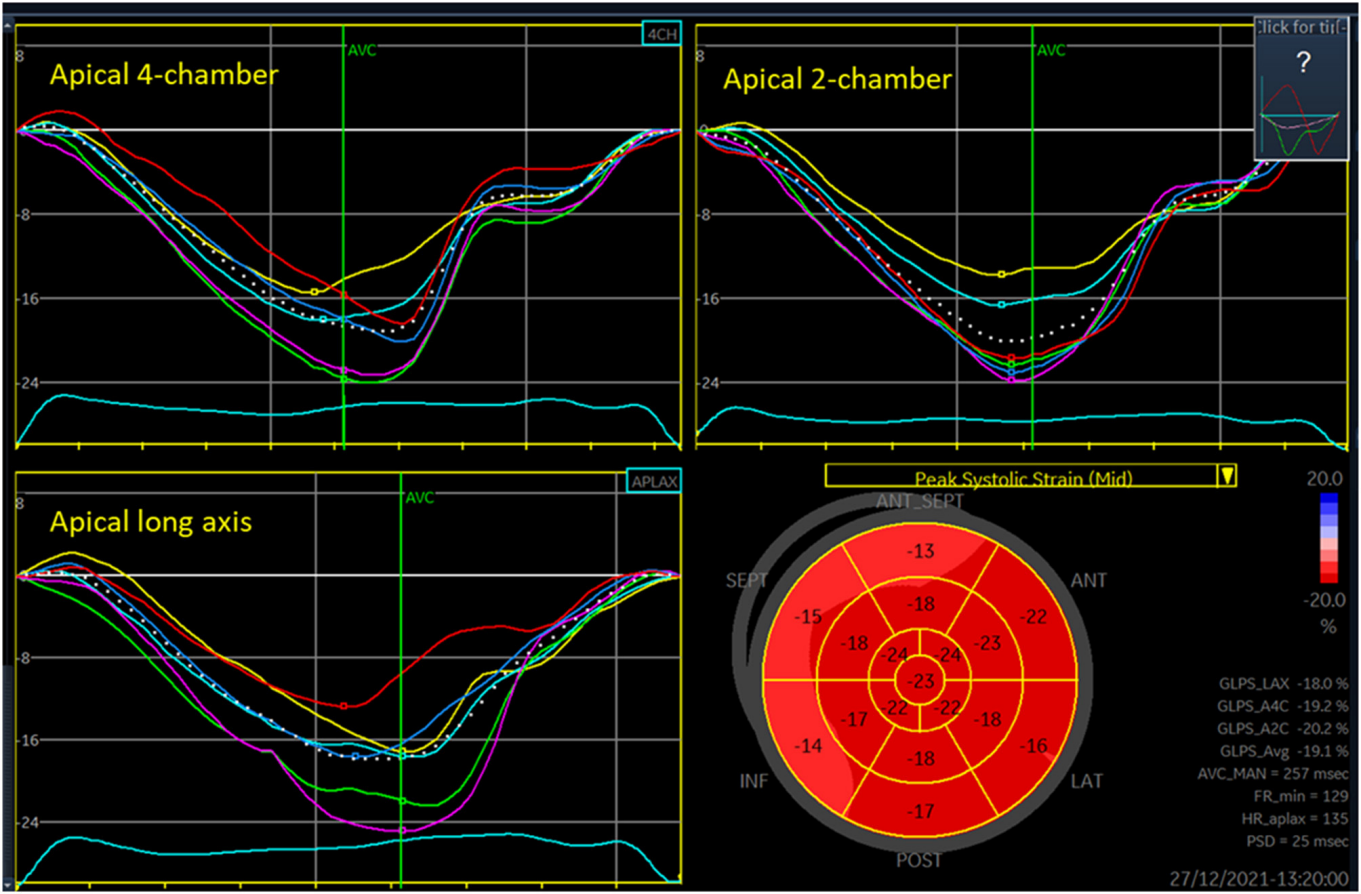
Three-plane longitudinal strain analysis of the left ventricle. Each of the panels for the three views shows seven curves, one colored curve from each of the six segments analyzed in each view and a white dotted curve for the entire region of interest. The low right panel shows values for each segment in a 17-segment plot, with basal segments at the outer edge and apical segments at the center of the figure (“bullseye plot”). The peak of each of the dotted curves is denoted GLPS (global longitudinal peak strain) for LAX (long axis), A4C (apical four-chamber), and A2C (apical two-chamber) views. The GLPS_Avg is the average of these three peaks, often referred as the global longitudinal strain. FR_min is the lowest grayscale frame rate (Hz) among the three views analyzed. HR_aplax is the heart rate in the apical long axis recording (beats per minute). PSD is the standard deviation of the time from onset of systole to segmental peak strain for each of the segments, often denoted as mechanical dispersion.
